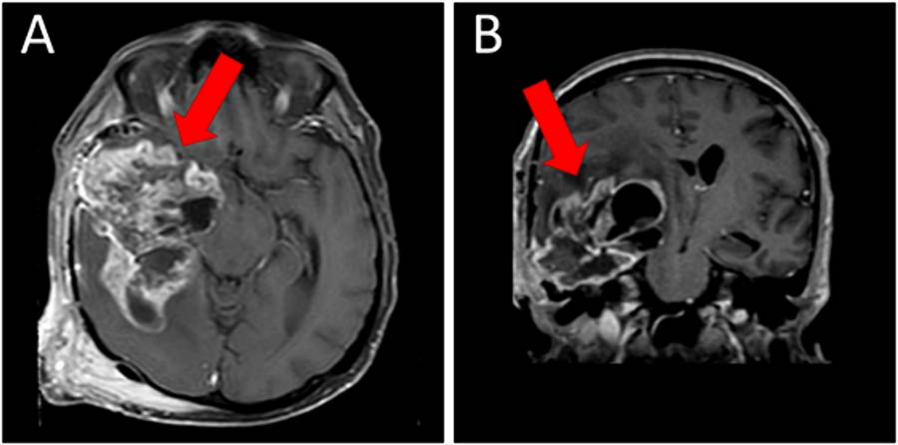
Preoperative T1WI (A) axial and (B) sagittal MRI images of a huge right temporo-parietal intra-axial lesion (indicated by arrows), which show enhancement after gadolinium injection. The axial image also shows extracranial subgaleal plexiform neurofibroma. MRI = magnetic resonance imaging, T1WI = T1-weighted images
